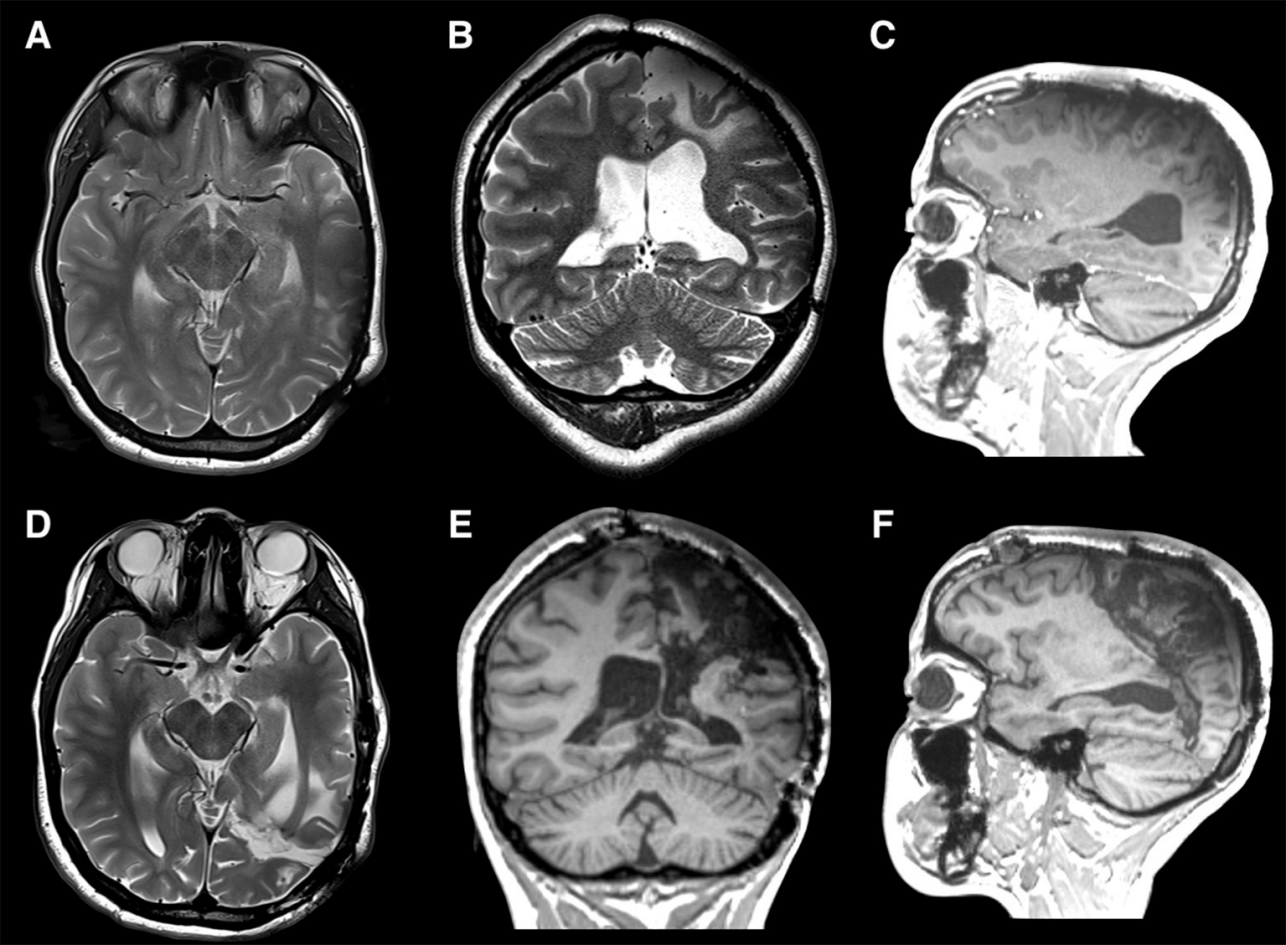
An 18-year-old left-handed girl presented with medically refractory epilepsy that was symptomatic since the age of 3 years due to a perinatal stroke in the territory of the left middle cerebral artery. Cerebral MRI (multiplanar) confirmed the radiological sequelae of an ischemic lesion involving primarily the central, parietal, and occipital lobes (A–C). At the age of 7 years, based on phase II subdural grid evaluation, the majority of the seizures were determined to arise from the supplementary motor area (SMA) on the left side for which she underwent a subpial resection of the SMA. She continued to have an unfavorable seizure outcome and regression of developmental milestones along with a mild right hemiparesis with a limited fine motor movement of the right hand. Phase I and II (SEEG) evaluations showed major epileptogenic foci in the left parasagittal parietal lobe and precentral area. When the centro-parieto-occipital lobes (central quadrant) are involved, in the presence of normal frontal and temporal lobes, the situation becomes complex for a curative surgical procedure. A precise knowledge of the tracts that could traverse the lines of disconnection is mandatory. The association fibers like the SLF I, II, and III, IFOF, MLF, and cingulum are to be sectioned in addition to disconnecting the occipital lobe from the functional temporal lobe. The corticospinal, thalamocortical, parieto-pontine, occipito-pontine and optic radiations represent the projection fibers disconnected. The posterior lateral extension of the anterior commissure, tapetum, posterior third of corpus callosum, and splenium are the commissural fibers that are disconnected in this surgery. In view of the refractory nature of the epileptic illness and progressive cognitive decline, we decided to perform a centro-parieto-occipital lobotomy (central quadrantotomy). During this lobotomy, the arcuate fasciculus was spared, by performing a posteriorly directed oblique white matter section trajectory at the posterior insular point. Indeed, the direction of this disconnective procedure follows the direction of the forces that cause the telencephalic flexure during the early embryological development. The language functions remained unchanged and similar to the pre-operatively acquired language skills. The patient remained in Engel class I at the last follow-up at 10 months after surgery. Postoperative MRI (multiplanar) demonstrated the complete disconnections (arrows) of the central quadrant with preservation of the AF (D–F).
